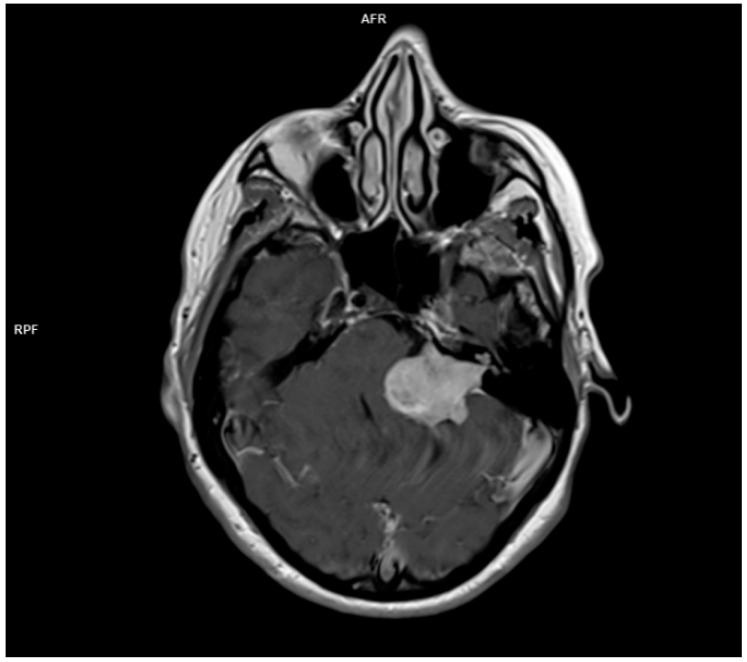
Axial T1-weighted contrast-enhanced MRI acquired at 3 Tesla (Siemens Magnetom Lumina, Siemens Healthcare S.R.L., Bucharest, Romania) with a 1 mm slice thickness. The image shows a large left vestibular schwannoma (acoustic neuroma) occupying the internal auditory canal and extending into the cerebellopontine angle, causing compression of the pons and left cerebellar hemisphere.
